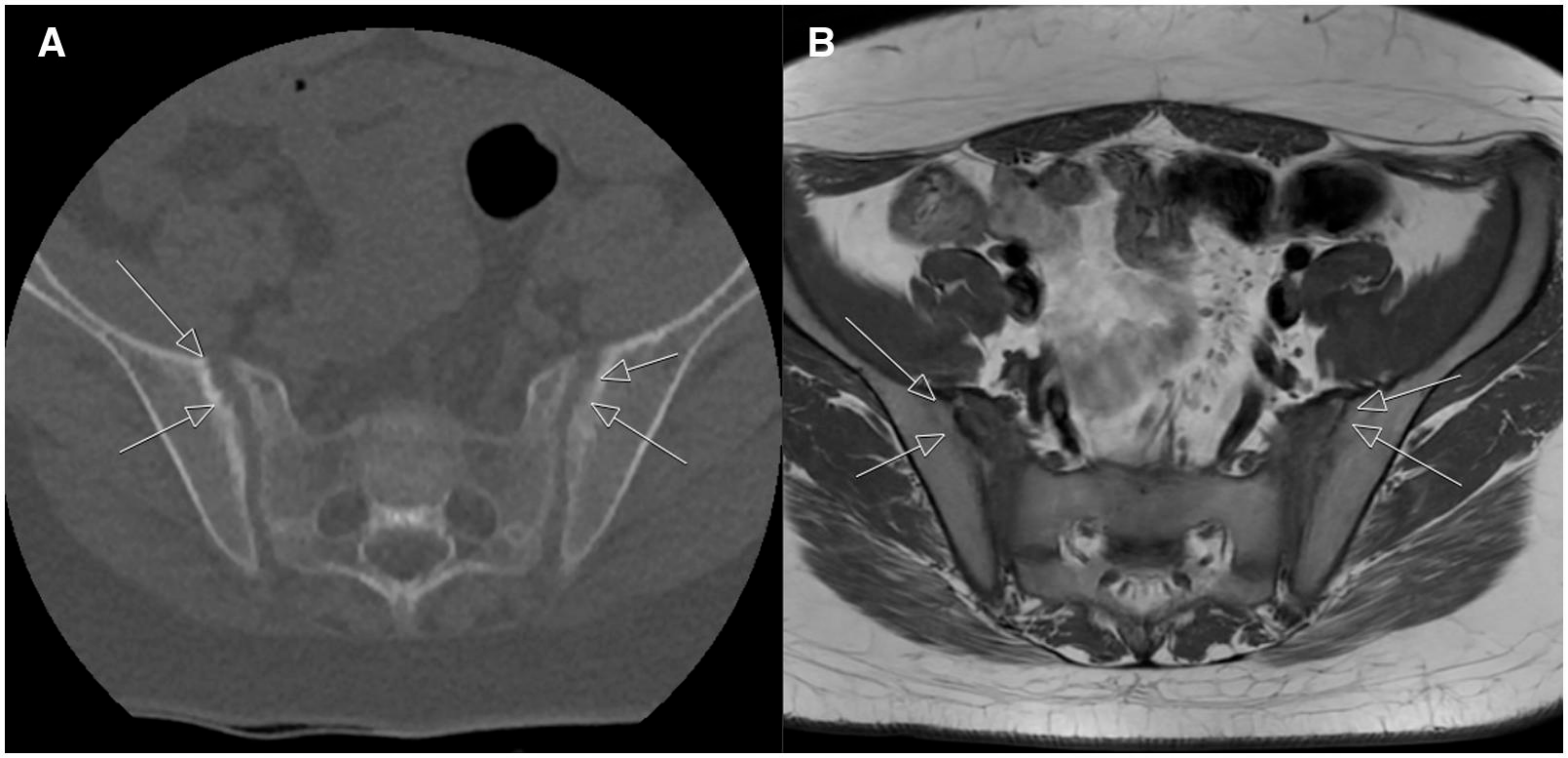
Widespread millimetric erosion is evident on CT, whereas it appears as millimetric erosion on MRI. In the axial section (A) of the low-dose CT scan of the sacroiliac joint, numerous millimetric-sized erosion areas, accompanied by sclerosis, are observed on both iliac surfaces (indicated by white arrows). In the axial non-contrast T1-weighted MRI section (B), barely visible millimetric erosions (white arrows) are noted on bilateral iliac face. When comparing sacroiliac joint images obtained from the same patient and at similar levels, erosion is much more clearly visualized on CT. In addition, many of the erosion visible on CT cannot be observed on MRI. Figures emphasizes that while the erosion is noticeable on MRI, it is clearly visible on CT.
